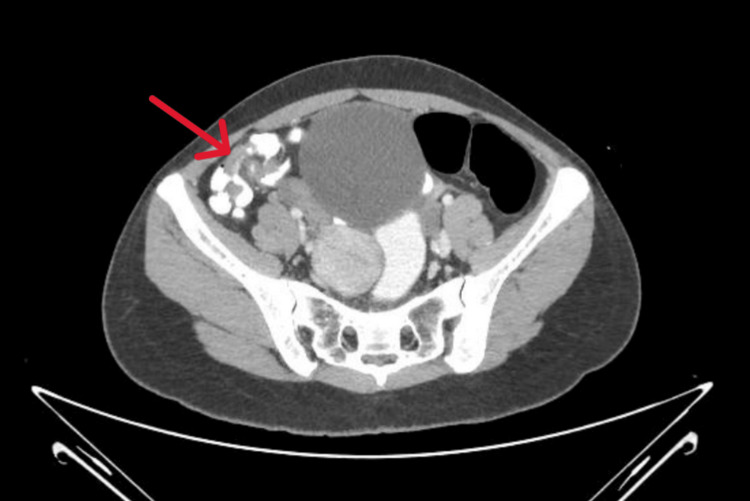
Contrast-Enhanced CT Demonstrating Mild Uncomplicated Appendicitis (Case 3) Axial CECT scan shows a mildly dilated appendix (red arrow) with heterogeneous wall enhancement and minimal periappendiceal fat stranding. No appendicolith or abscess is seen, consistent with early uncomplicated appendicitis.
